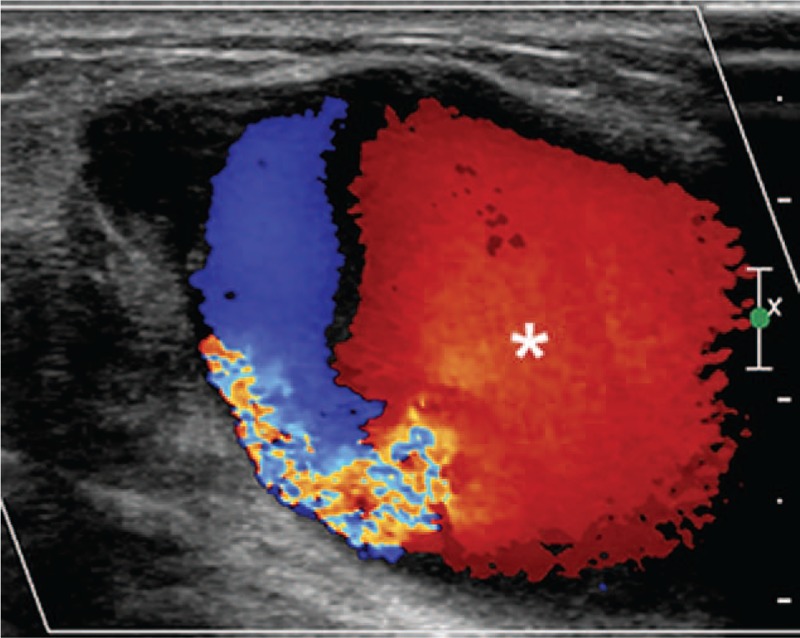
Color Doppler imaging revealed an anechoic mass with typical whirling blood flow and a red and blue, biphasic “to-and-fro” pattern, suggesting pseudoaneurysm (asterisk).
